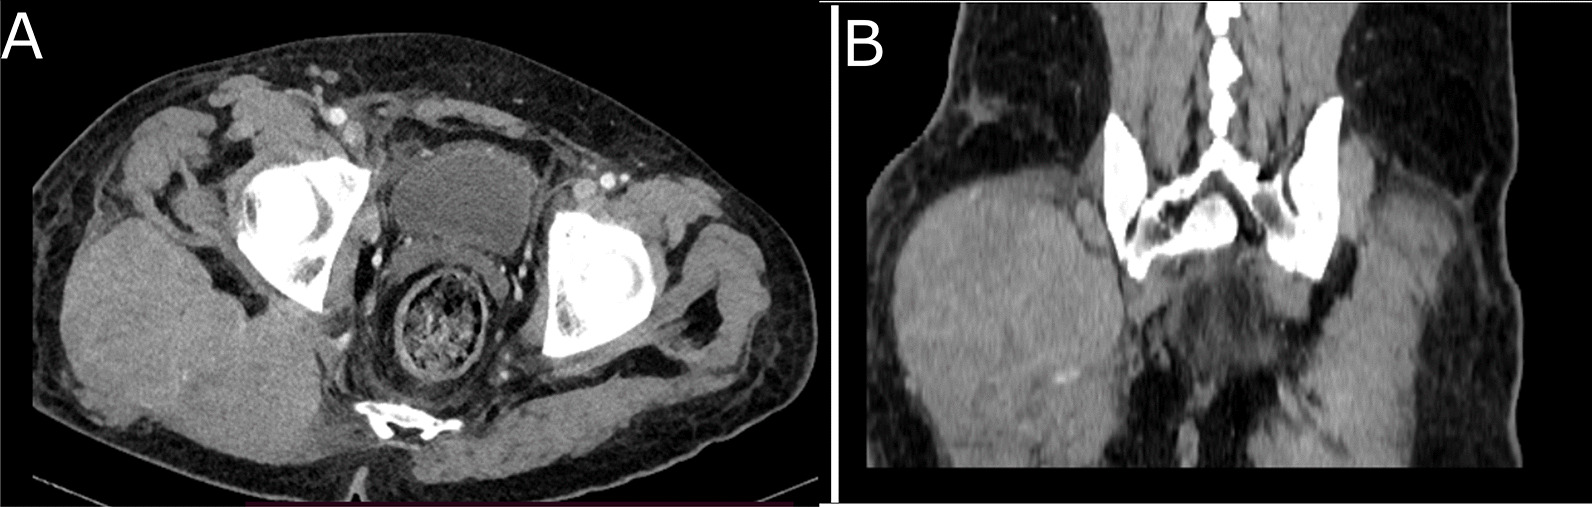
CT scan in axial (A) and coronal (B) sections. Mass at the expense of the gluteus maximus muscle
